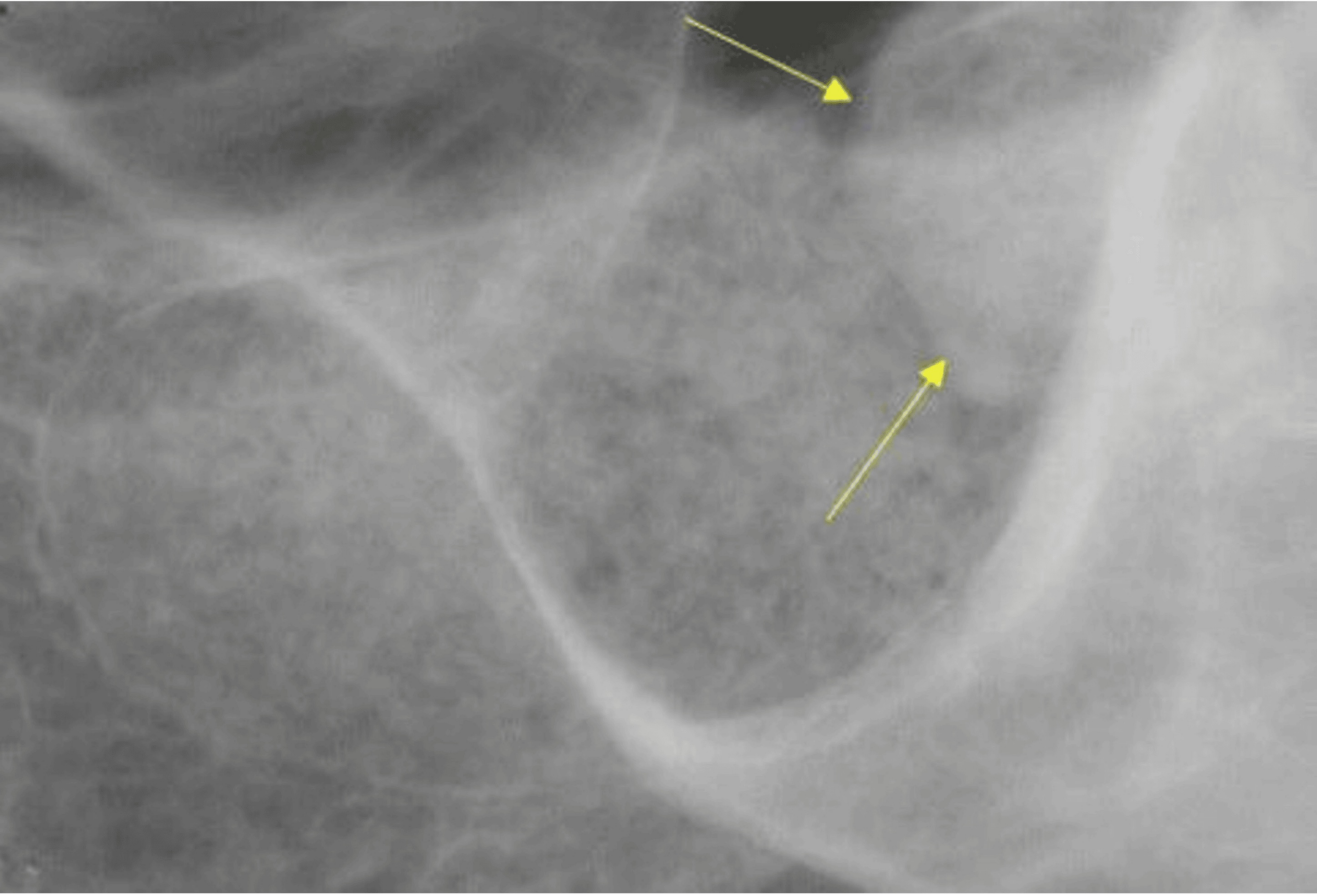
Intraoral periapical (PA) radiograph of the area of missing #14 and #15, acquired in 2024 at the one-month follow-up after the sinus augmentation procedure. The yellow arrows show the high-density entity under investigation.
